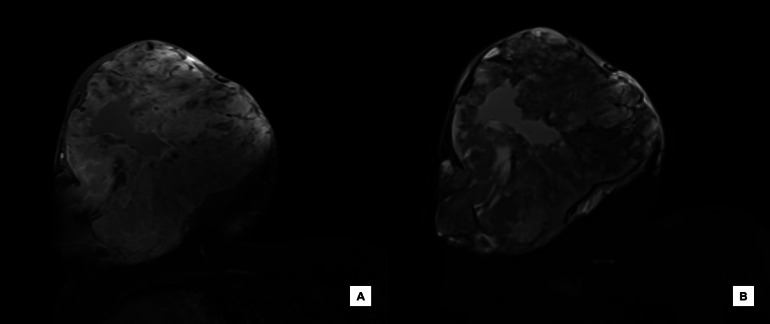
(A) Axial T1-weighted postcontrast fat saturated MRI image of the right breast demonstrating a 3.1 cm mass with avid, heterogenous enhancement and areas of intermediate signal intensity suggestive of hemorrhage and (B) Axial T2-weighted MRI image of the same mass demonstrating variable signal intensity.
